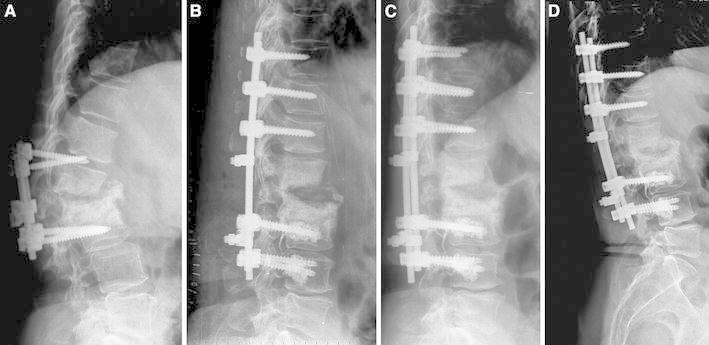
P.A., 57 years. a Failure of previous surgery performed in another hospital. b Postoperative X-ray. c, d Follow-up X-rays taken three and six months after treatment, respectively
